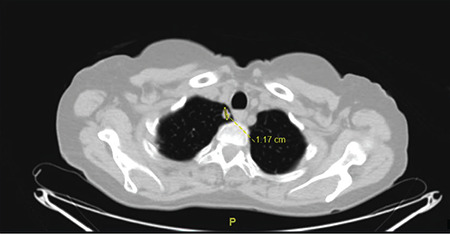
CT scan showed right upper lung lesion in diameter of 1,2*1,2 cm CT: Computed tomography
